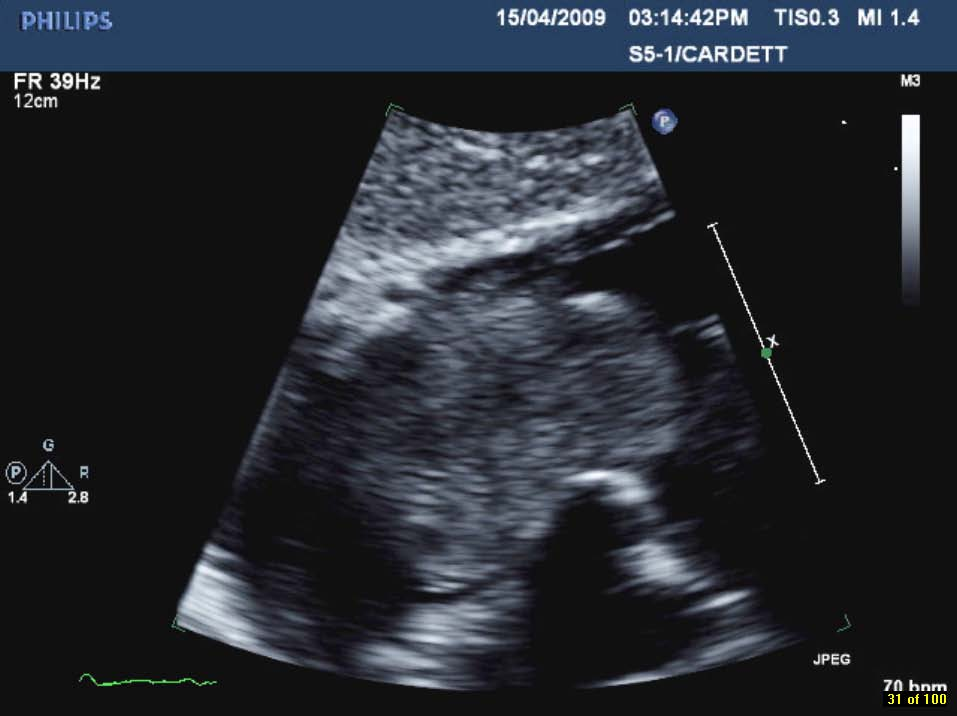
Right atrial mass on 2D-echocardiography. A subcostal view showing a huge echogenic mass in the right atrium, prolapsing through the tricuspid valve.
